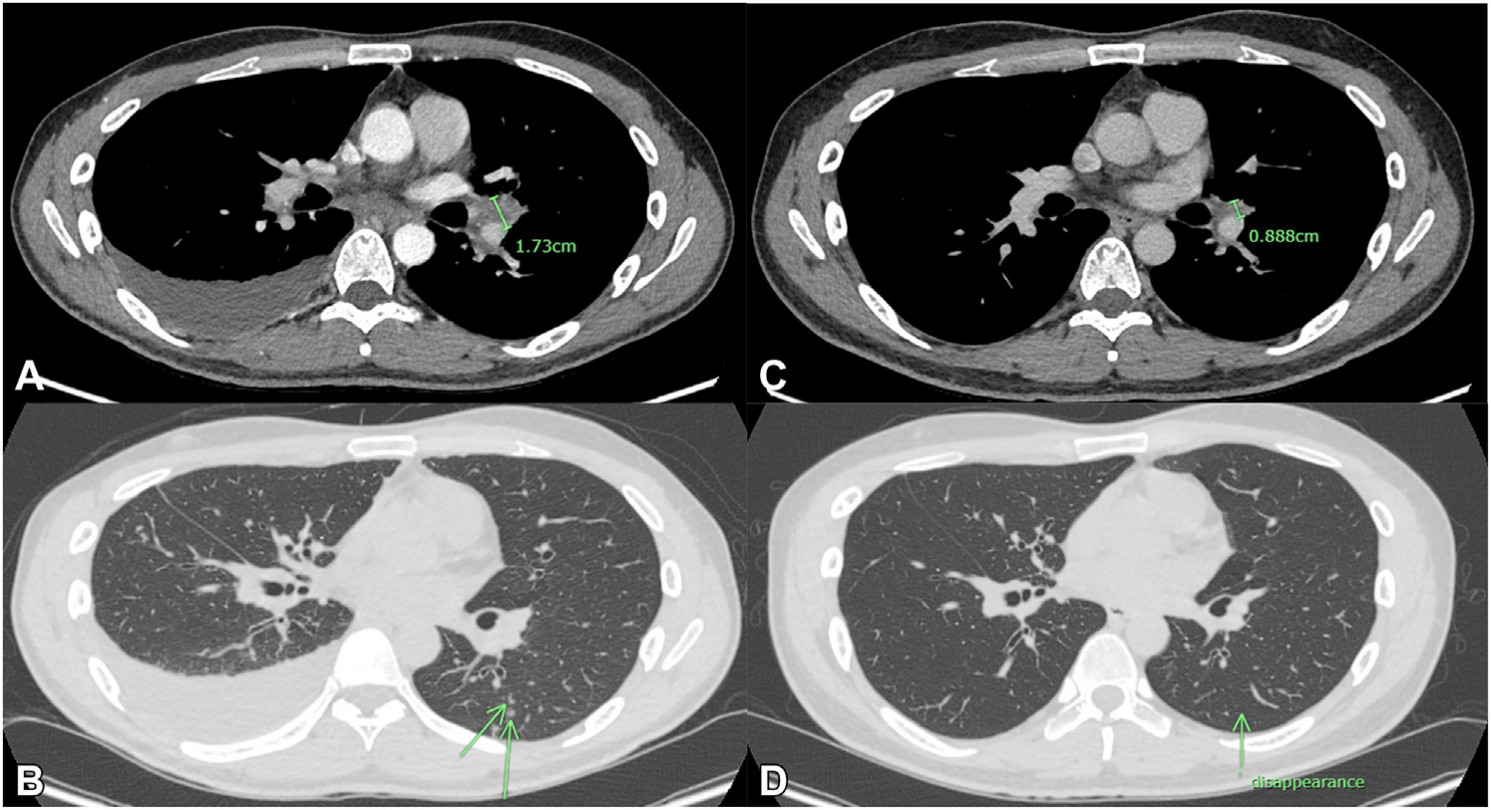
Chest computed tomography demonstrated. A, Right pleural effusion with hilar lymphadenopathy at baseline. B,*Arrow* shows multiple areas of centrilobular nodules in a tree-in-bud pattern. C, Pleural effusion was resolved, and the size of the hilar lymph node significantly decreased after a complete course of antituberculosis treatment. D, Pulmonary nodules disappeared.
